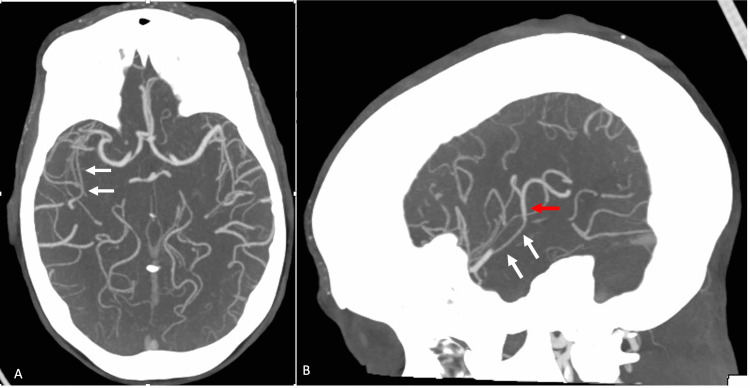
Axial and sagittal maximum intensity projection (MIP) post-contrast CTA images of the head Axial (A) and sagittal (B) maximum intensity projection (MIP) post-contrast CTA images of the head demonstrate a narrowed posterior division M2 branch of the right MCA (white arrows). There is a sharp transition with reconstitution of the normal caliber of the vessel distally (red arrows).
